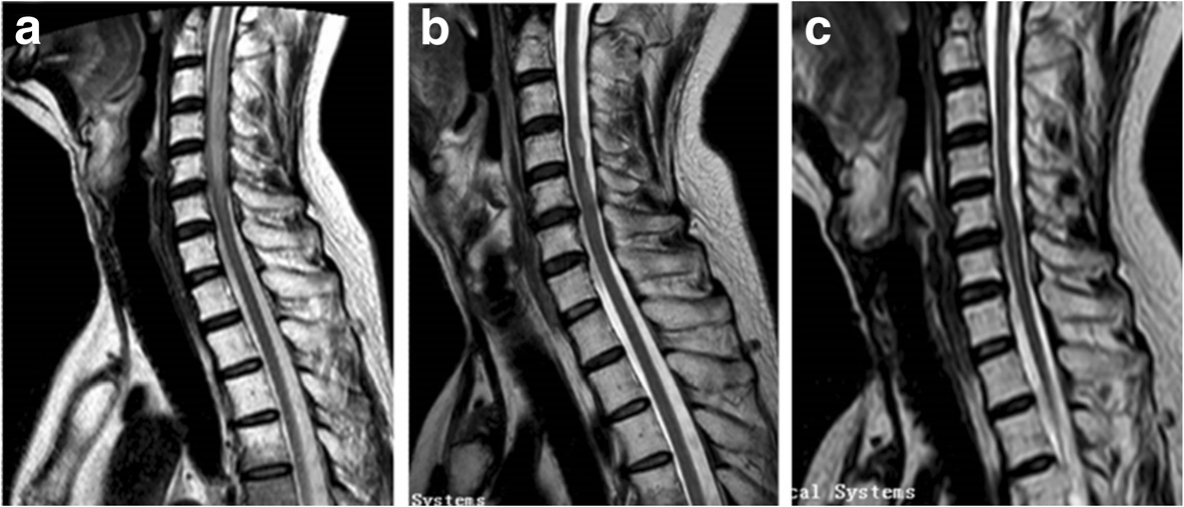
Comparison of MRI T2-weighted images of the spinal cord. a MRI before UC-MSCT revealing fresh linear hyperintensities within the swollen cervical cord from C4 to C6. b At 6-month follow-up, MRI demonstrated obvious regression of the previous lesions. Swelling of the spinal cord was reduced significantly. c At 18-month follow-up, MRI did not show new enhanced lesions in the spinal cord
